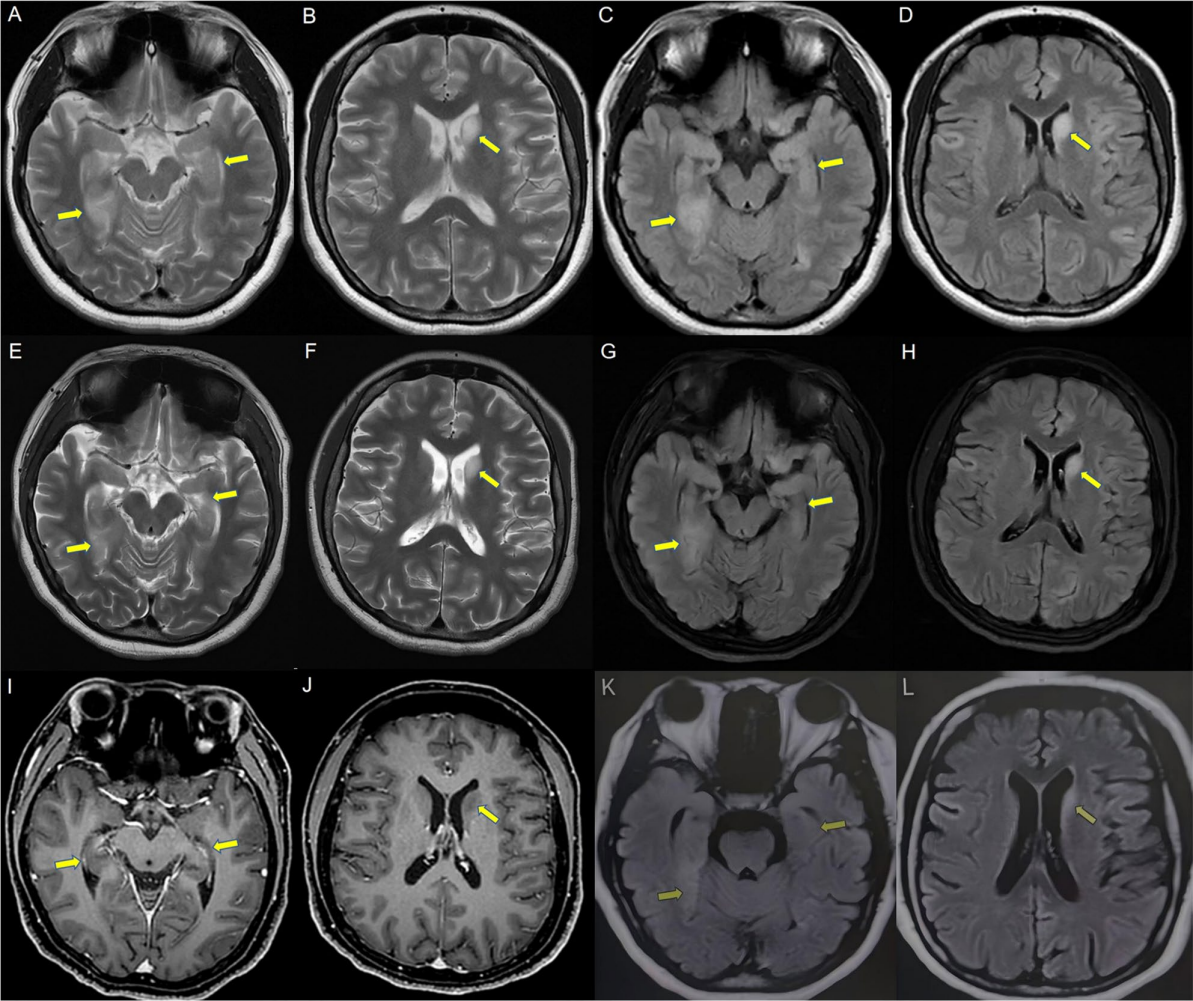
Brain MRI findings of patient No.1. A ~ D After symptom onset, increased T2WI and T2-fluid-attenuated inversion recovery (FLAIR) signal could be observed in bilaterally medial temporal lobe and hippocampus, right caput nuclei caudati. E ~ J Follow-up imaging 7 days later, the range of the T2WI and T2-FLAIR hyperintensity decreased slightly. Contrast image showed no enhancement. K, L Follow-up imaging 20 days later, the range of hyperintensity decreased significantly
